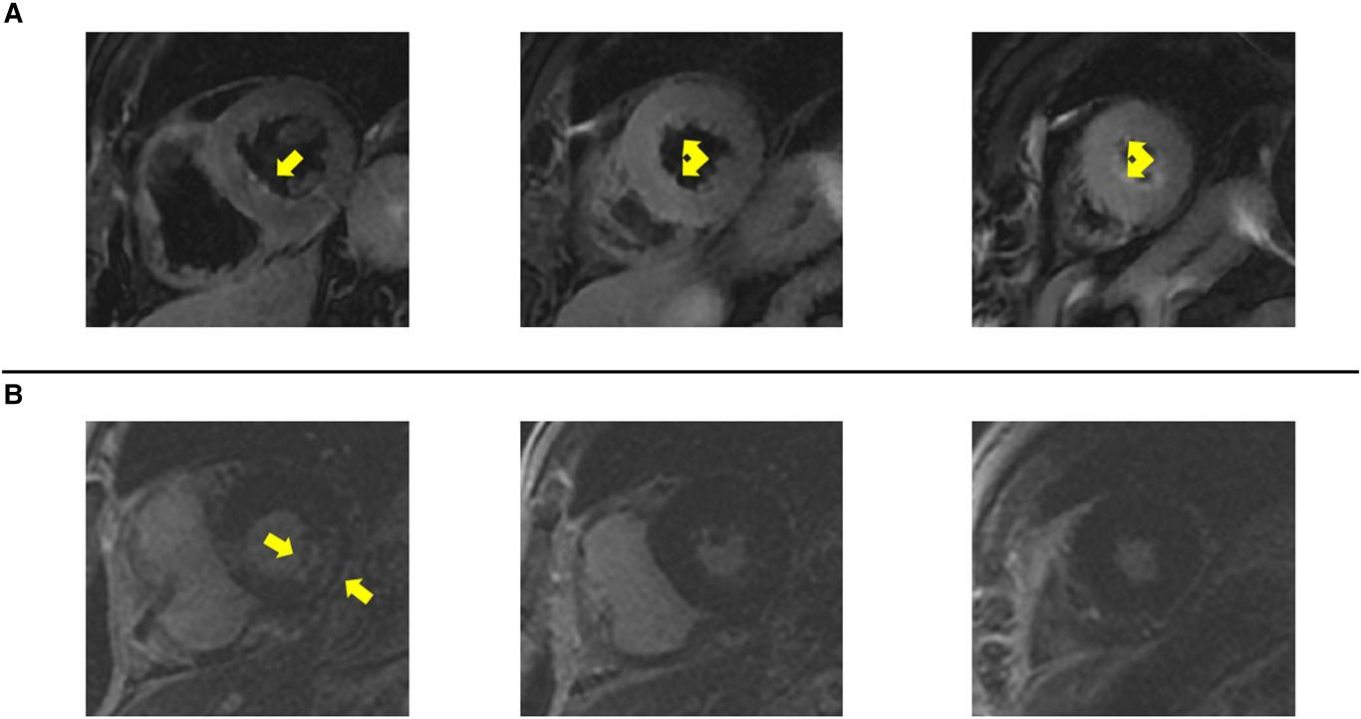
Cardiac magnetic resonance T2-weighted short-tau inversion recovery sequences (short-axis views; *A*) show diffuse transmural oedema (arrows) involving the apical and mid-segments of the septum, anterior, and inferior walls, as well as subendocardial oedema of the basal infero-lateral wall. T1-weighted gradient echo-inversion recovery sequences (short-axis views; *B*) show late gadolinium enhancement (arrows) limited to the basal infero-lateral wall.
